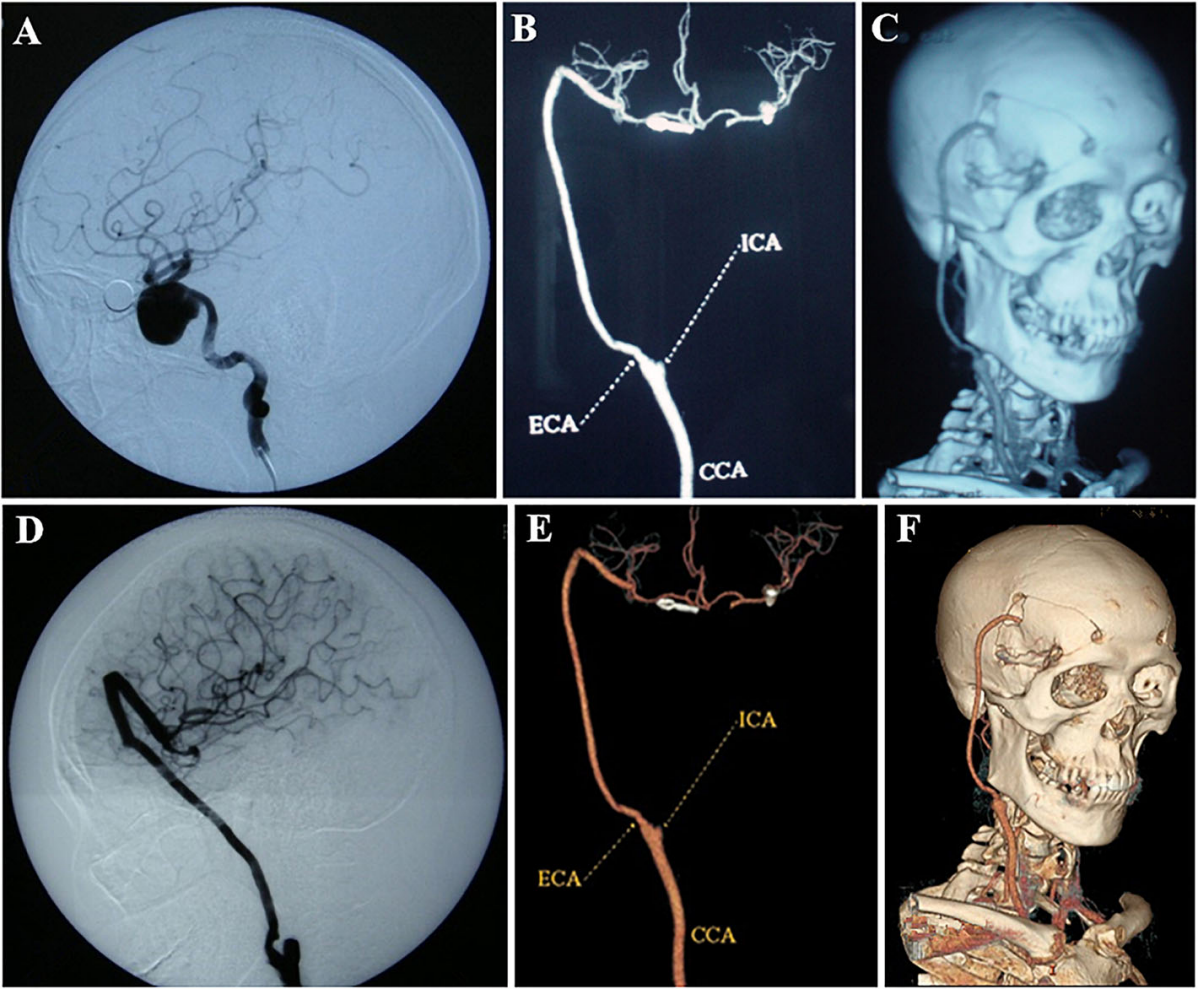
Preoperative, postoperative and follow-up imaging results. A: preoperative DSA can find a huge complex intracranial aneurysm in cavernous sinues; B, C: CTA results on the first day after operation and before discharge, we found that the aneurysm had disappeared and the bypass vessel was unobstructed.; D, E, F: DSA results at 6 months follow-up showed good
